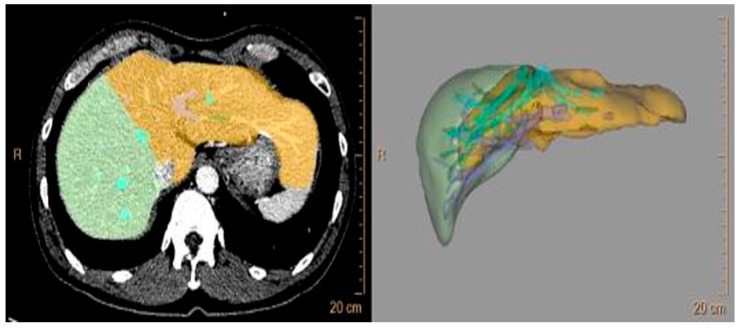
Liver volumetry. Three-dimensional reconstruction of the liver was rendered with preoperative liver dynamic computed tomography (CT) using a software program (TeraRecon Acuaris iNtuition version 4.4.12).
